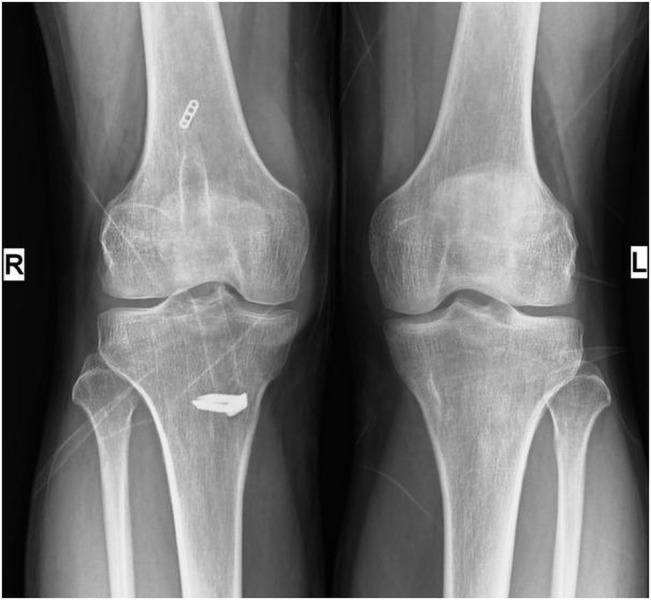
AP radiographs of the knees showing tunnel widening in the right femur and tibia after ACLR
